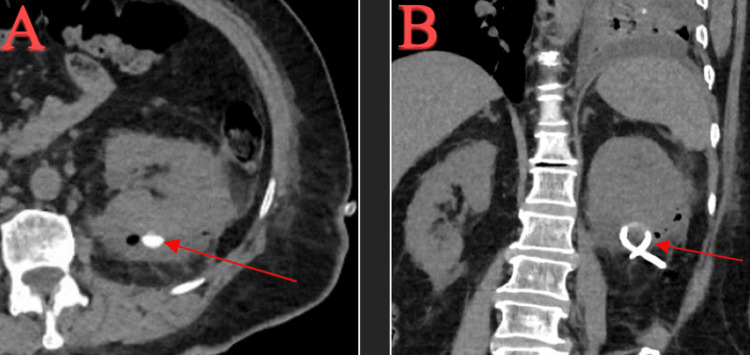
CT abdomen and pelvis w/o contrast of the left kidney subcapsular hematoma with a percutaneous drain in place: (A) transverse view and (B) coronal view. CT, computed tomography
